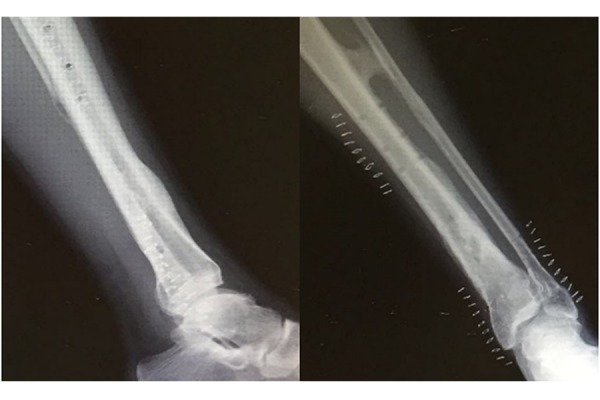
X-ray shows the removal of plates and screws after 1 year from the injury
